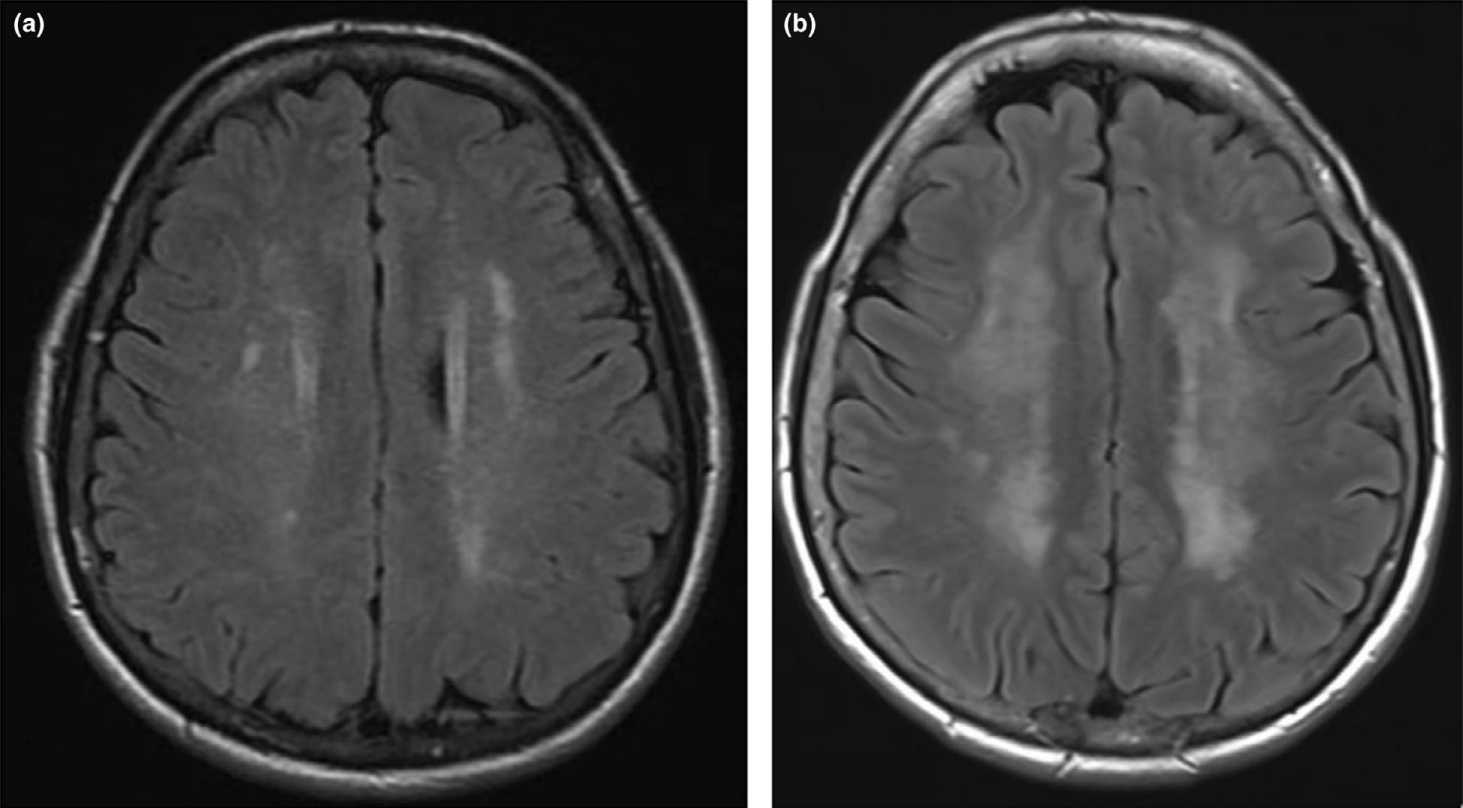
Axial FLAIR MRI brain in a 61‐year‐old female patient with GPA showing moderate amount of supratentorial deep white matter FLAIR hyperintensity in keeping with small vessel vasculopathy (a). This white matter ischaemia markedly progressed as compared to the previous MRI 5 years prior to this examination (b).
